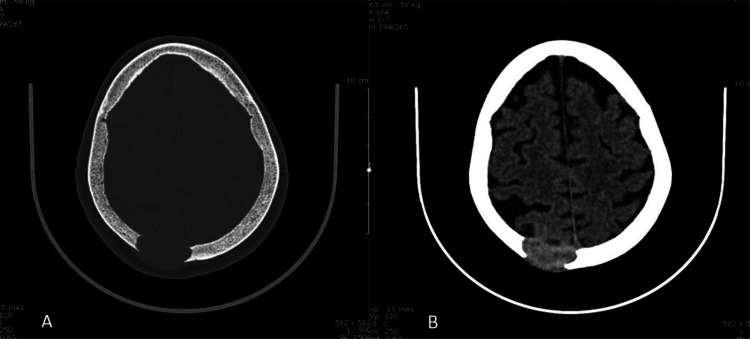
Cerebral CT axial section in bone window (A) and parenchymal window (B). Presence of a right parieto-occipital lytic bone lesion measured in its long axis 34 mm. Involvement of the periosteum and meningeum, without pial or cortical passage, is noted.
